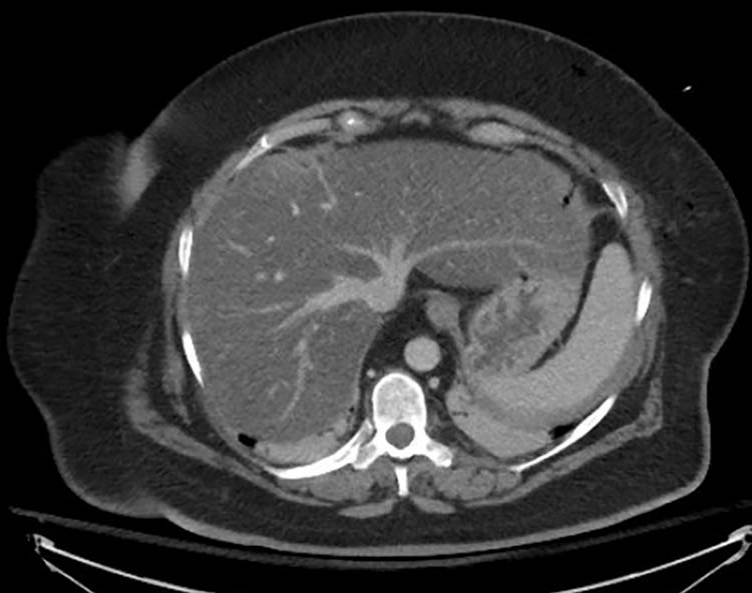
CT thorax and abdomen, infused, immediately post-operative noting severe fatty infiltration within the liver and Veress needle track.
